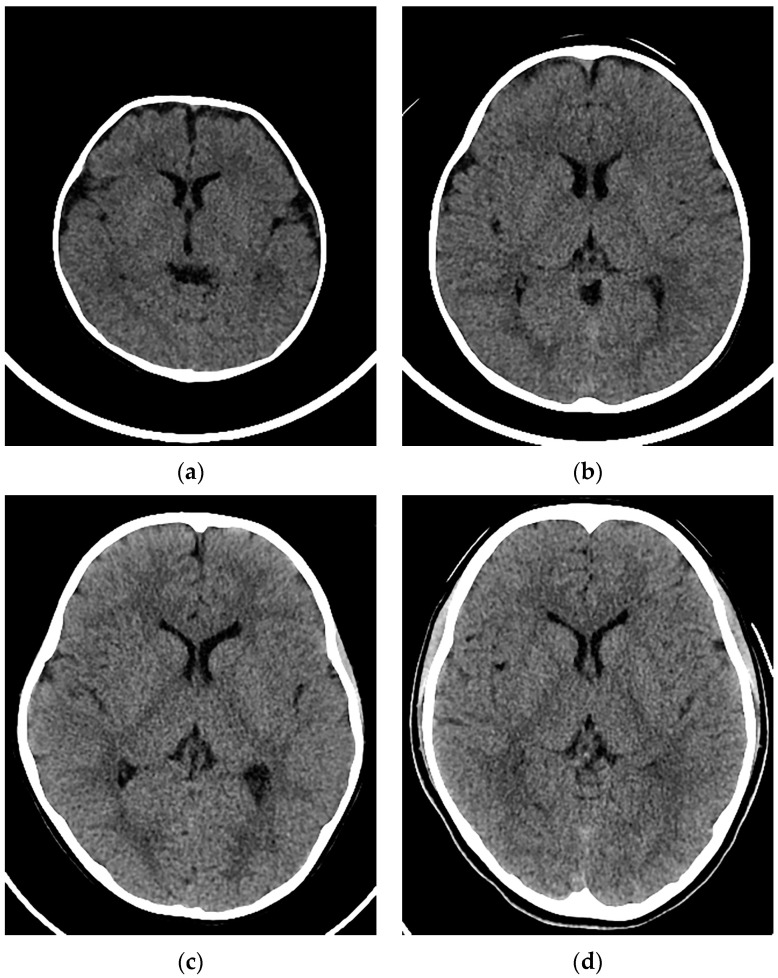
Examples of CT images in a (a) 3-month-old girl, (b) 1-year-old boy, (c) 5-year-old girl, and (d) 10-year-old girl.
